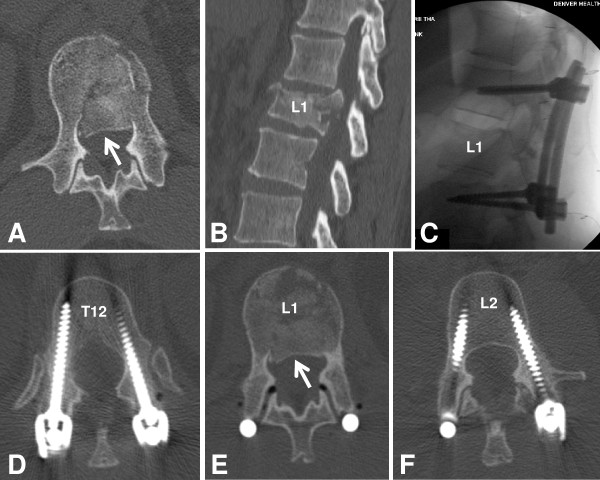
Clinical example of "spine damage control" for an unstable L1 complete burst fracture (AO/OTA 53-A3.3) with 50% spinal canal narrowing (A, B) in a 50-year old lady who sustained an axial loading trauma mechanism in a commercial airliner crash at Denver International Airport. The patient was neurologically intact (ASIA grade E). She was taken for a posterior reduction and two-level instrumentation T12-L2 on day 1. Intraoperative fluoroscopy films show an excellent reduction of the L1 burst fracture with restoration of near-anatomic vertebral body height and sagittal profile in lordosis (C). The postoperative CT scan (D-F) demonstrates a significant clearance of the anterior spinal canal fragment by pure ligamentotaxis (arrow in E, compared to arrow in A). The patient tolerated the operative procedure well. She was mobilized on postoperative day (POD) #1 with physical therapy and discharged on POD #2 to fly back to her hometown, where she followed up with a local spine surgeon for anterior completion fusion. This example emphasizes the safety and efficacy of the "spine damage control" concept.
